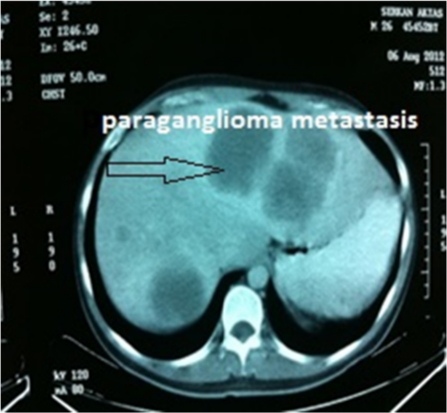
CT image of paraganglioma at the sixth months.
